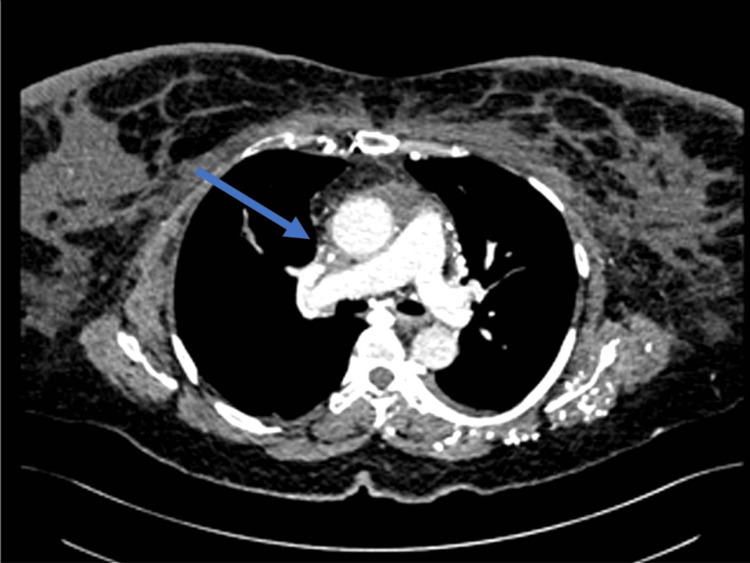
Computed tomography of the chest with contrast showing a filling defect in the superior vena cava at the level of the tracheal bifurcation.
